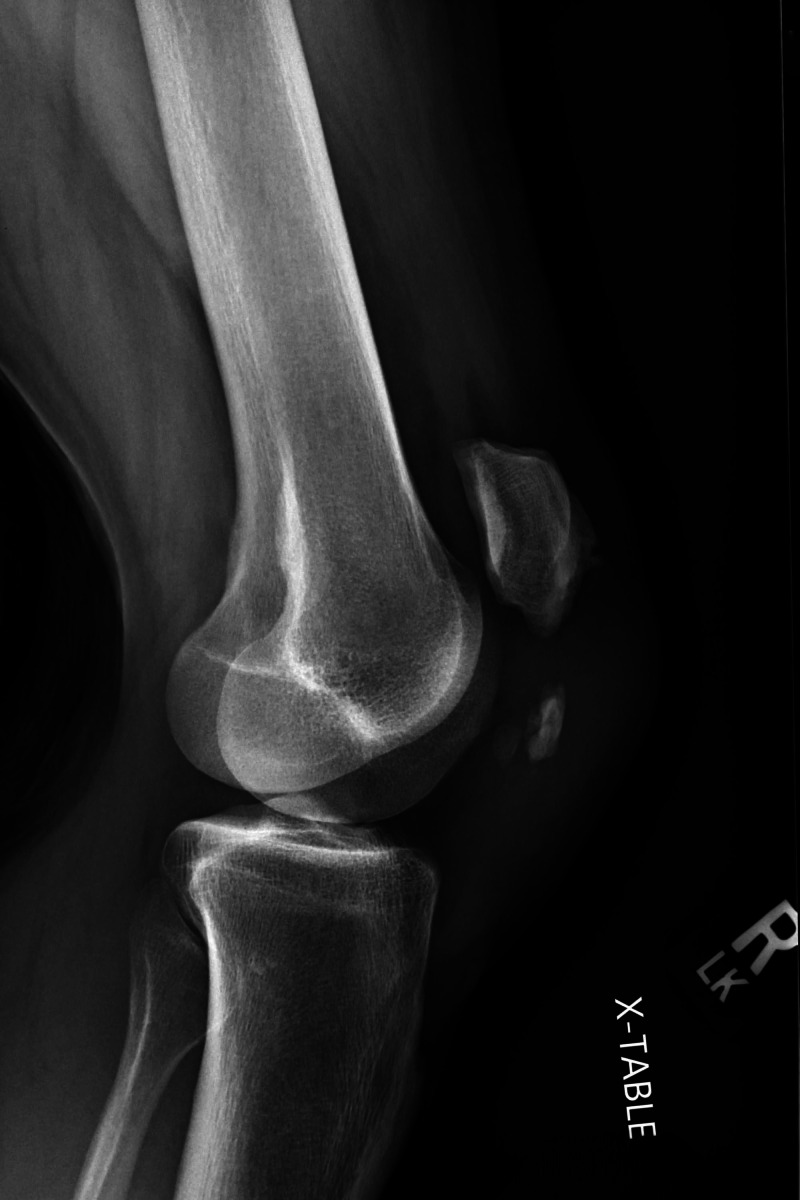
Lateral view radiograph of the right knee
